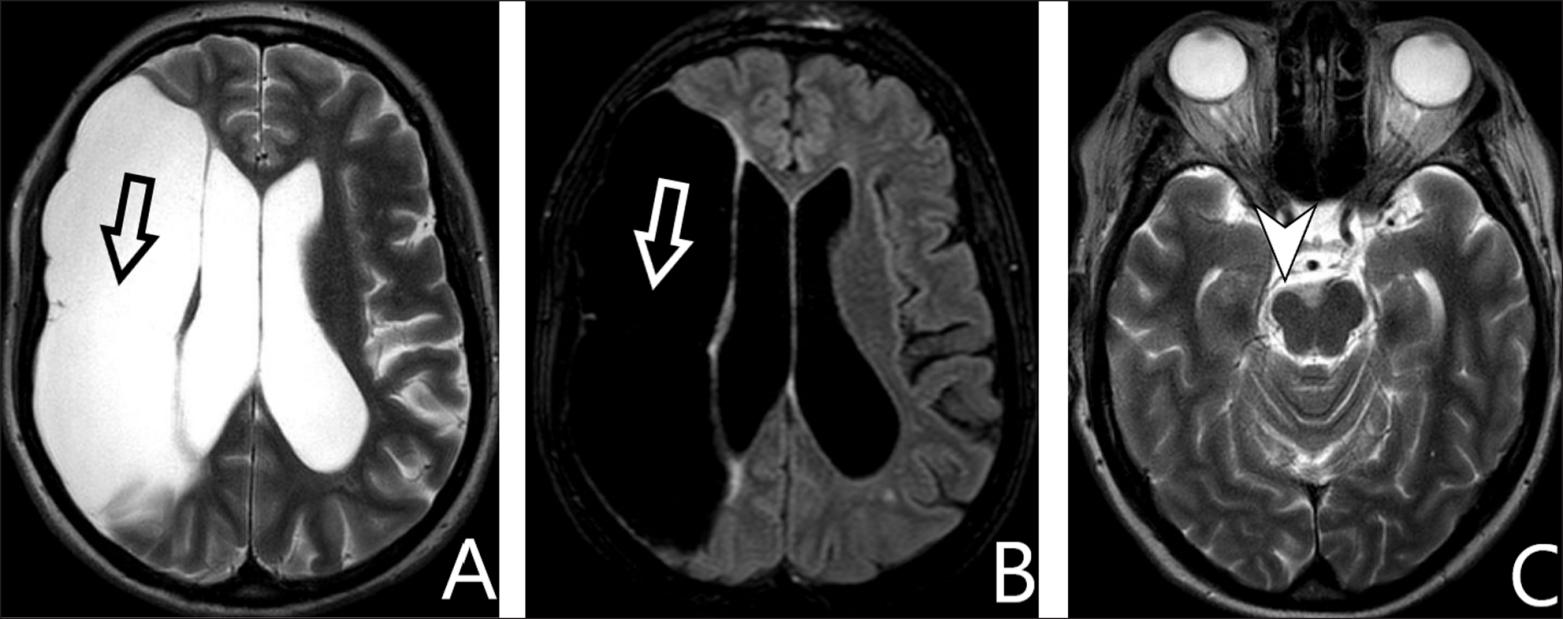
A: *axial T2-WI*, B: *FLAIR*, C: *T2-WI*. Right frontoparietal large arachnoid cyst, hyperintense on T2-WI and hypointense on FLAIR (*arrows*). Atrophy of the right cerebral peduncle (*arrowhead*).
